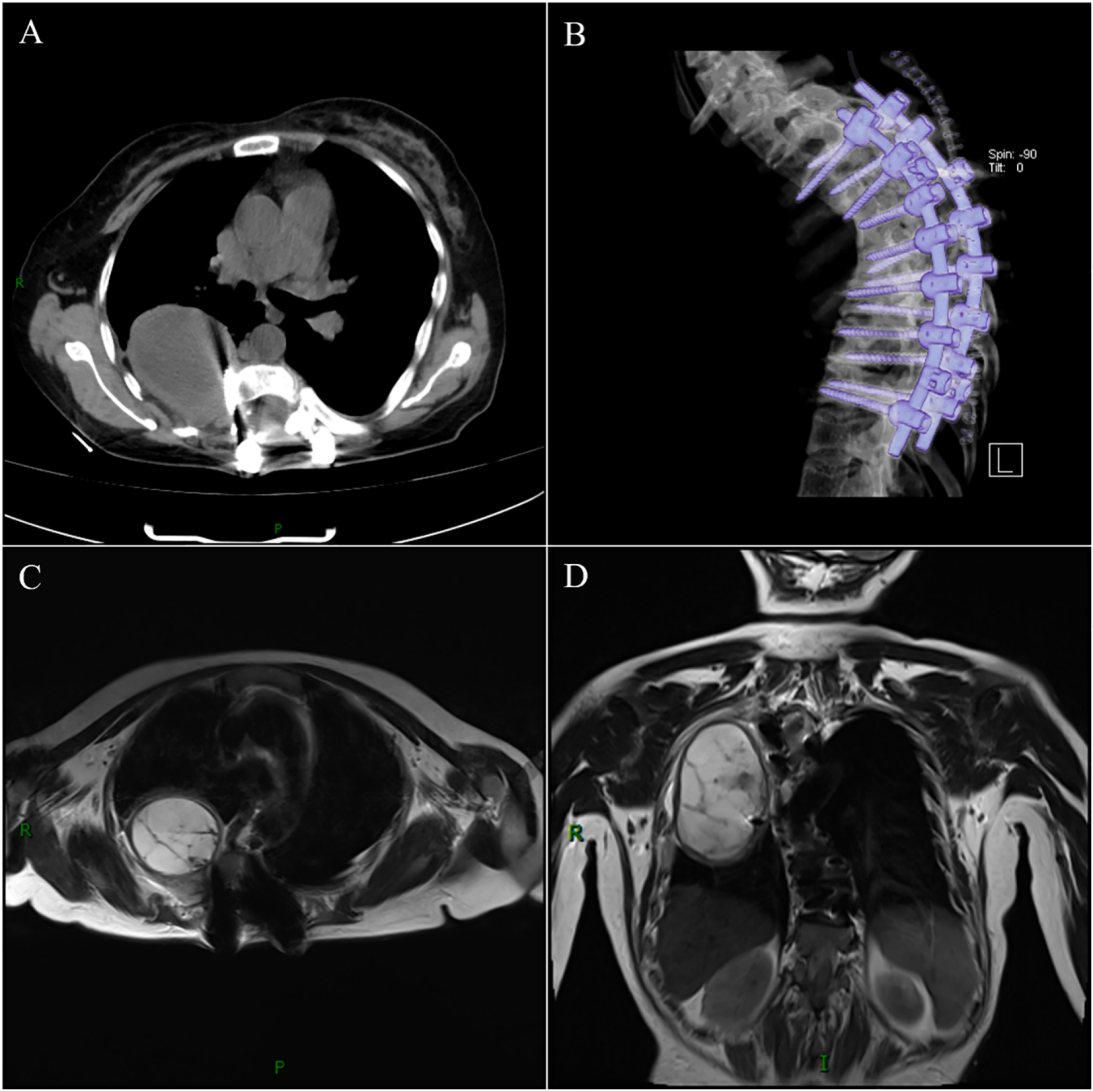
Initial postoperative chest CT: Demonstrates a residual cystic lesion in the right thoracic cavity at T5/T6 level with significant volume reduction compared to preoperative scans. Note well-positioned posterior titanium mesh grafting and T3-T9 pedicle screw fixation (A, B). Thoracic MRI at 3-month follow-up: Confirms stable size of the residual cystic lesion without interval growth and absence of recurrent pleural effusion (C, D).
